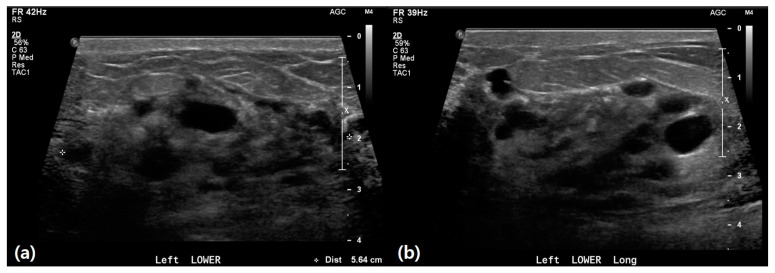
A 43-year-old female presented with erythema, a palpable mass, and pain in the breast. (a,b) Ultrasound imaging revealed heterogeneous echo texture in both breasts. The lower portion of the left breast exhibited diffuse skin thickening and edematous changes, with an indistinct heterogeneous hypoechoic lesion containing cystic components. These findings suggest a high likelihood of an infectious condition, such as mastitis. The patient was treated with medication, and clinical follow-up indicated improvement in symptoms.
